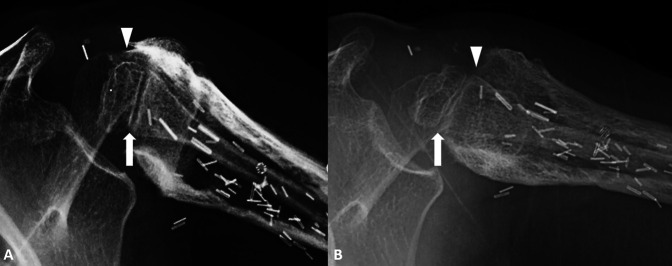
Axillary view radiographs show an open physis (arrow) with the tip of the epiphysis below the proximal portion of the allograft (arrowhead) at the 15-month follow-up period (A). At 27 months postoperative, the physis remained open (arrow) with the tip of the epiphysis now above the proximal portion of the allograft (arrowhead), highlighting the growth of the proximal humerus (B).
